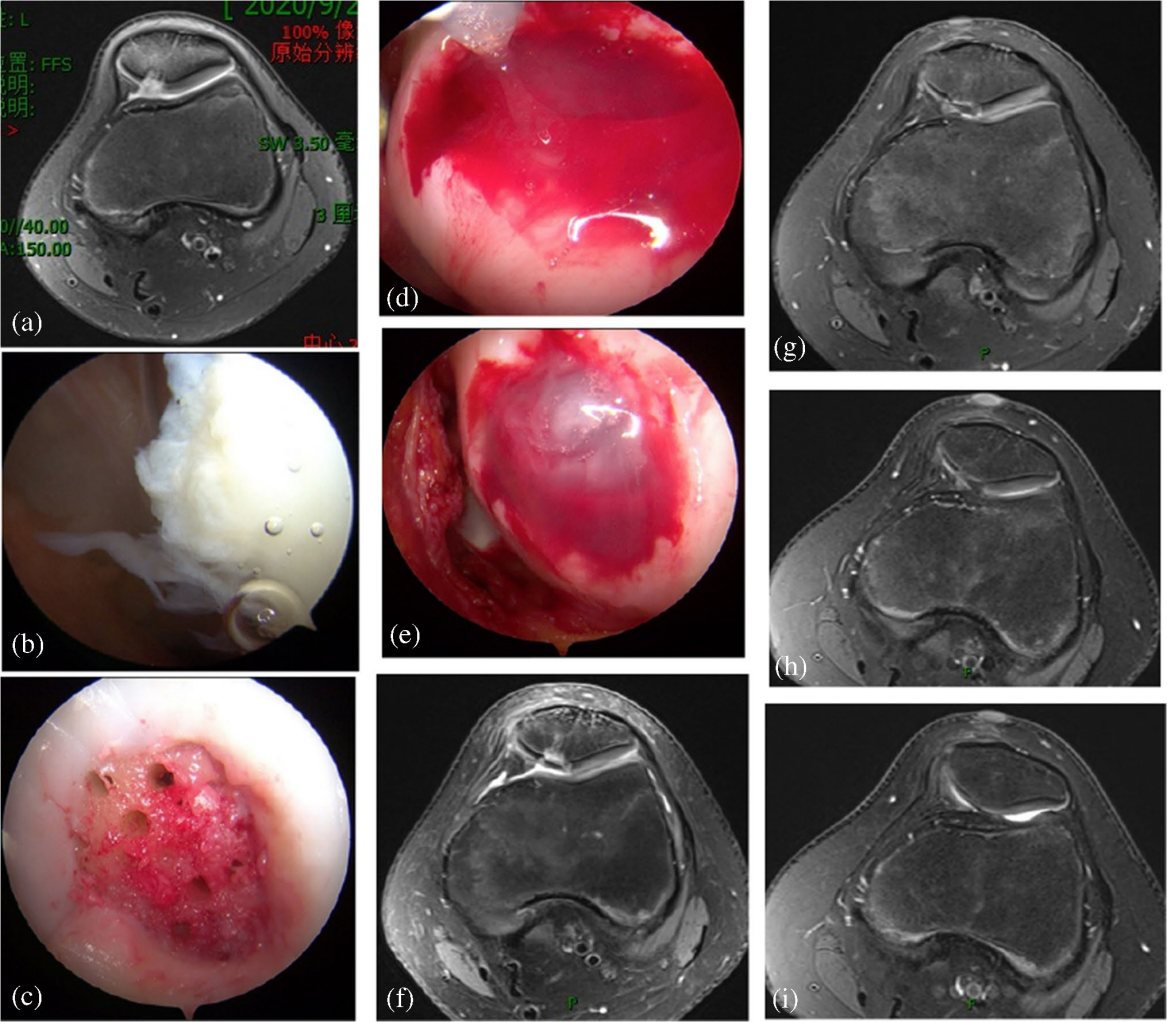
Images from typical cases. a The pre-operative MRI of the knee joint showed a full-thickness cartilage defect of the patella of approximately 2 cm^2^. b The full-thickness cartilage defect of the patella under arthroscopy. c A microfracture of the subchondral bone was created in the cartilage defect by using a microfracture device under arthroscopy. d Platelet-rich plasma and thrombin cover the microfracture wound. e The platelet-rich plasma and thrombin were sealed with fibrin gels. f Two months after surgery, the defects of the patella cartilage showed on the MRI. g Five months after surgery, the defects of the patella cartilage showed on the MRI. h Eight months after surgery, the defects of the patella cartilage showed on the MRI. i One year after surgery, the magnetic resonance imaging showed that most of the defects of the patella cartilage were repaired, and the area reduced to about 0.4 cm^2^
